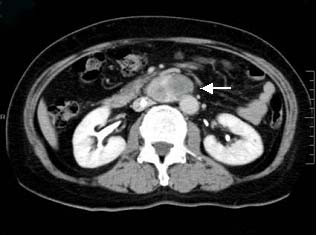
An abdominal contrast-enhanced CT scan incidentally demonstrated a heterogeneous mass, 6.5 cm in diameter, with cystic lesion in the retroperitoneum. The tumor exhibited marked enhancement after intravenous administration of contrast material.
